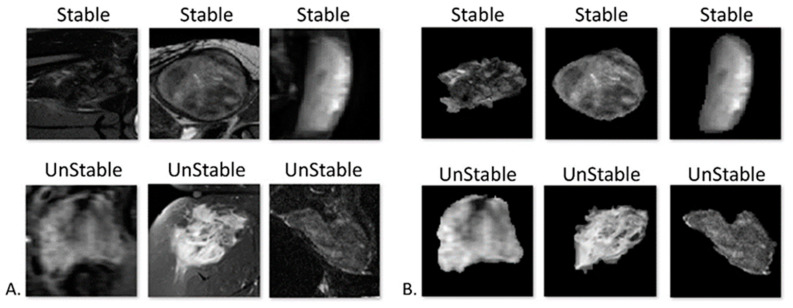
Input data. (A). Tumors with the background MRI sequence included. (B). Extracted 3D tumor model.
